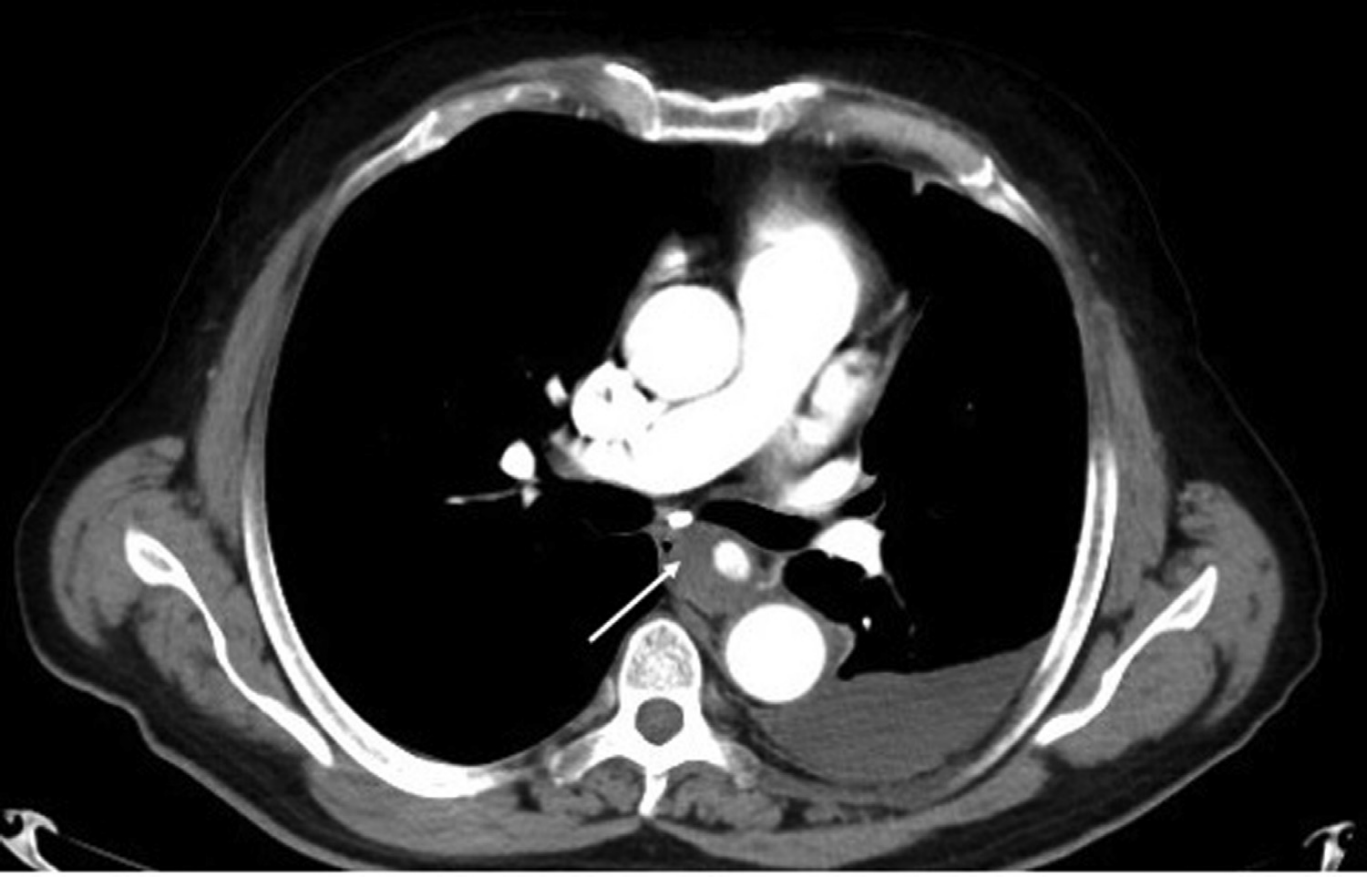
Axial CT scan view in the arterial phase showing a mediastinal mass enhancing the same as aorta (arrow). Notice the moderate pleural effusion (Liquid density 10 HU).
